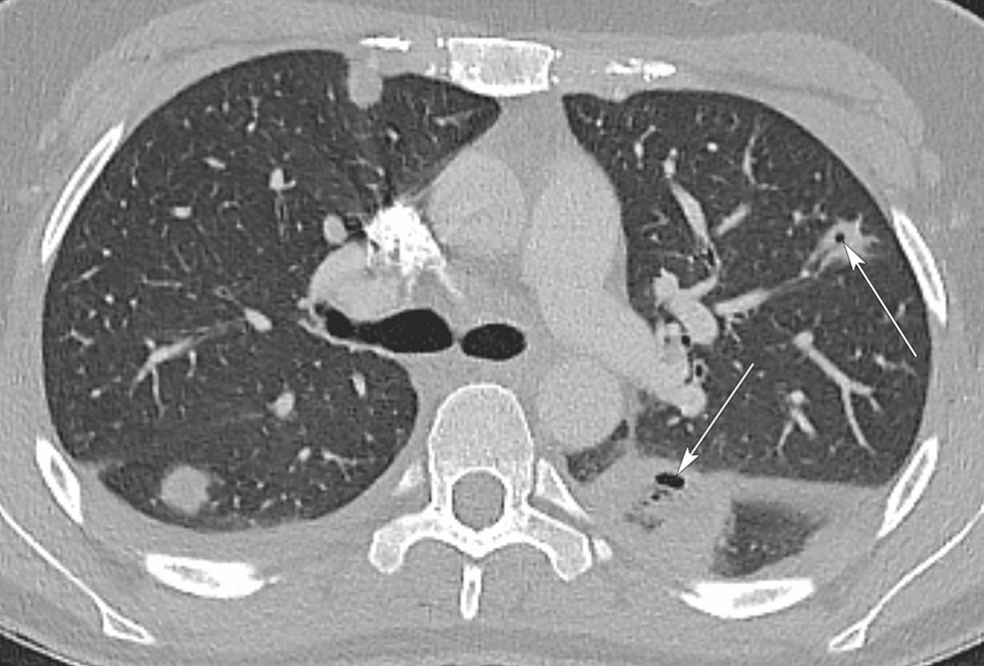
Axial CT pulmonary angiogram Axial CT image through the lungs at the level of the carina, lung window setting, showing multiple ill-defined soft tissue nodules in both lungs and sub-pleura. Multiple thick-walled lung cavitations can be seen (arrows), consistent with septic emboli.
